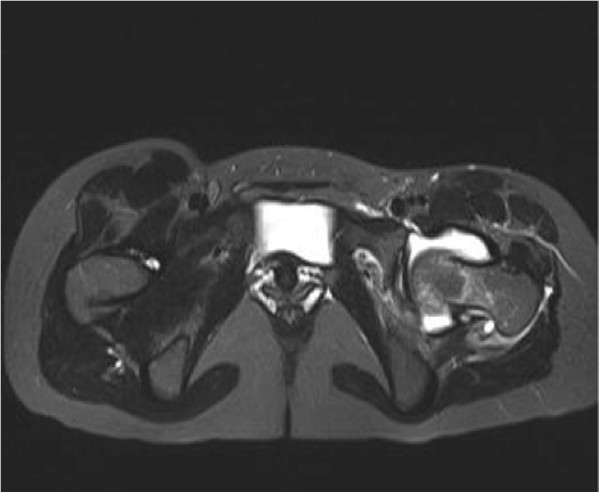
T2-weighted magnetic resonance imaging transverse plane of pelvis showed marked effusion over left hip joint.
